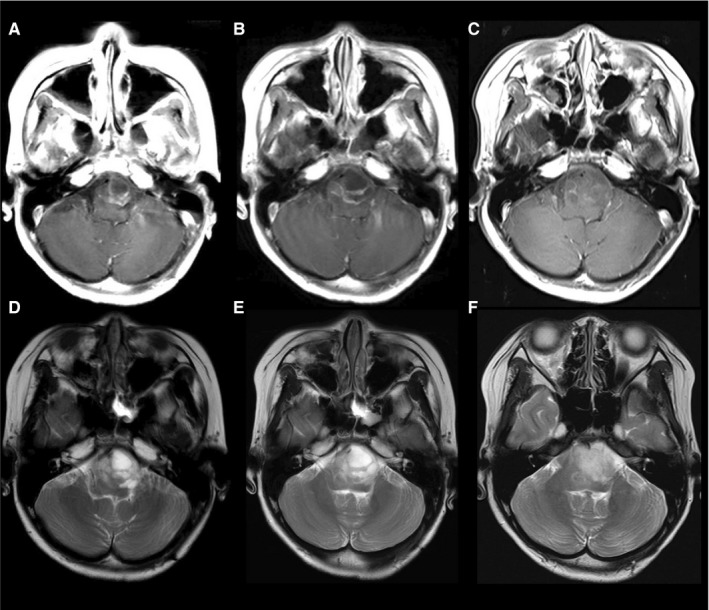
Gadolinium enhanced T1‐weighted imaging (A‐C) and T2‐weighted imaging (D‐F) of 12‐year‐old boy with recurrent diffuse midline glioma, H3 K27M‐mutant. One month before (A/D), immediately before (B/E), 1 mo after (C/F) bevacizumab (BEV) treatment. Cysts size increased before BEV (from A/D to B/E), while decreased after treatment with BEV (from B/E to C/F). However, T2/FLAIR high tumor size increased during both periods (from A/D to B/E, and from B/E to C/F). His dysphagia improved after BEV treatment
